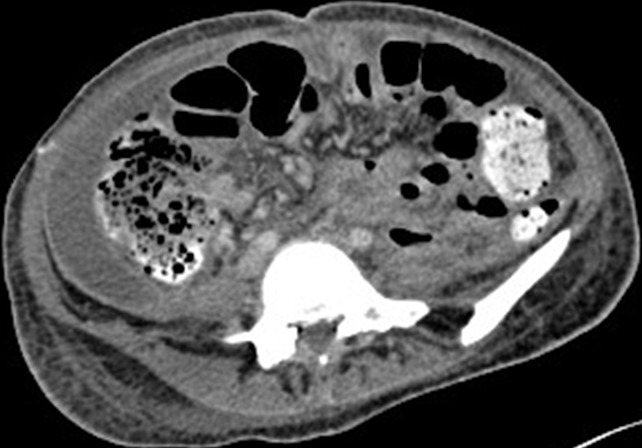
Axial CT scan after embolization and bilateral nephrectomy.
